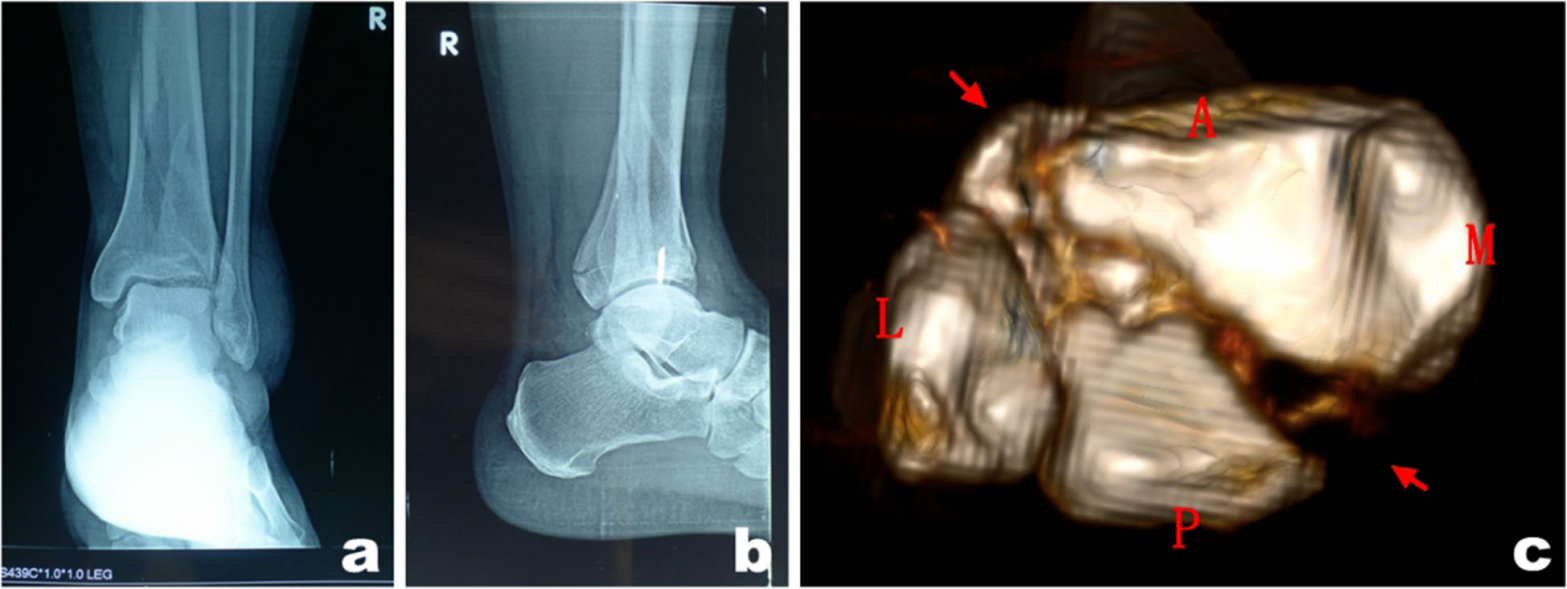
A 36-year-old male patient with Pilon fracture of the right tibia caused by fall injury. Preoperative X-ray (a, b) and CT scan (c) showed that the articular surface collapsed significantly, the posterior malleolar fragment involved the tarsal tunnel area, and the die-punch fragment was slightly anterolateral
